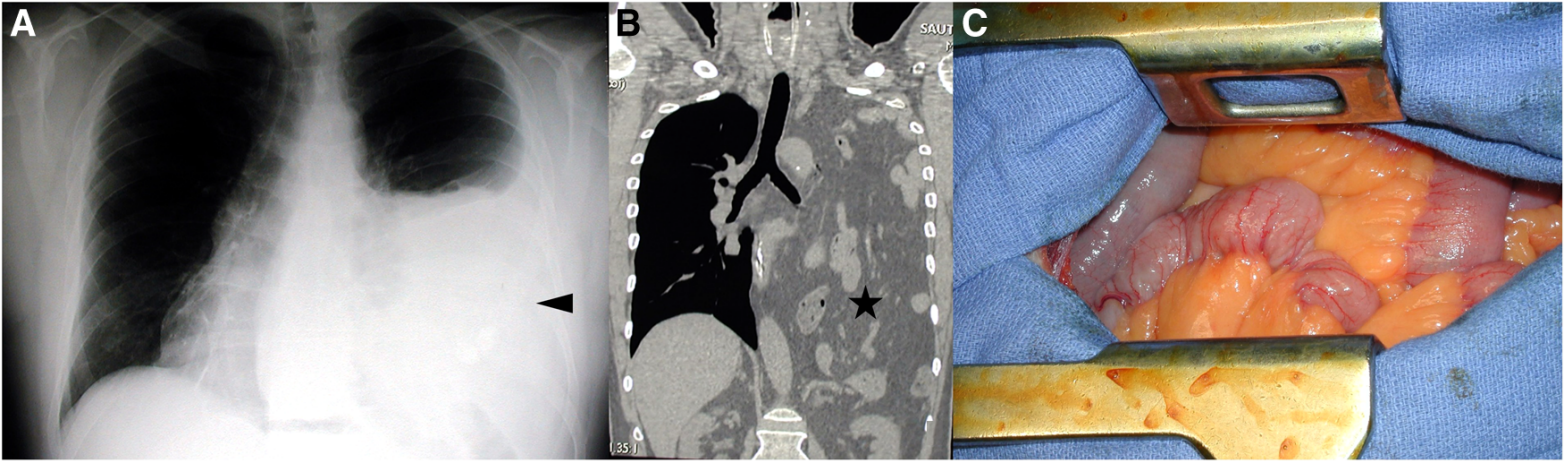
Case 1: (A) The thoracic x-ray is showing a left thoracic opacity up to the aortic arch; (B) the corresponding thoraco-abdominal CT in the frontal view showing the presence of intestinal content in the left thoracic cage; and (C) the herniated intestinal content following left thoracotomy.
